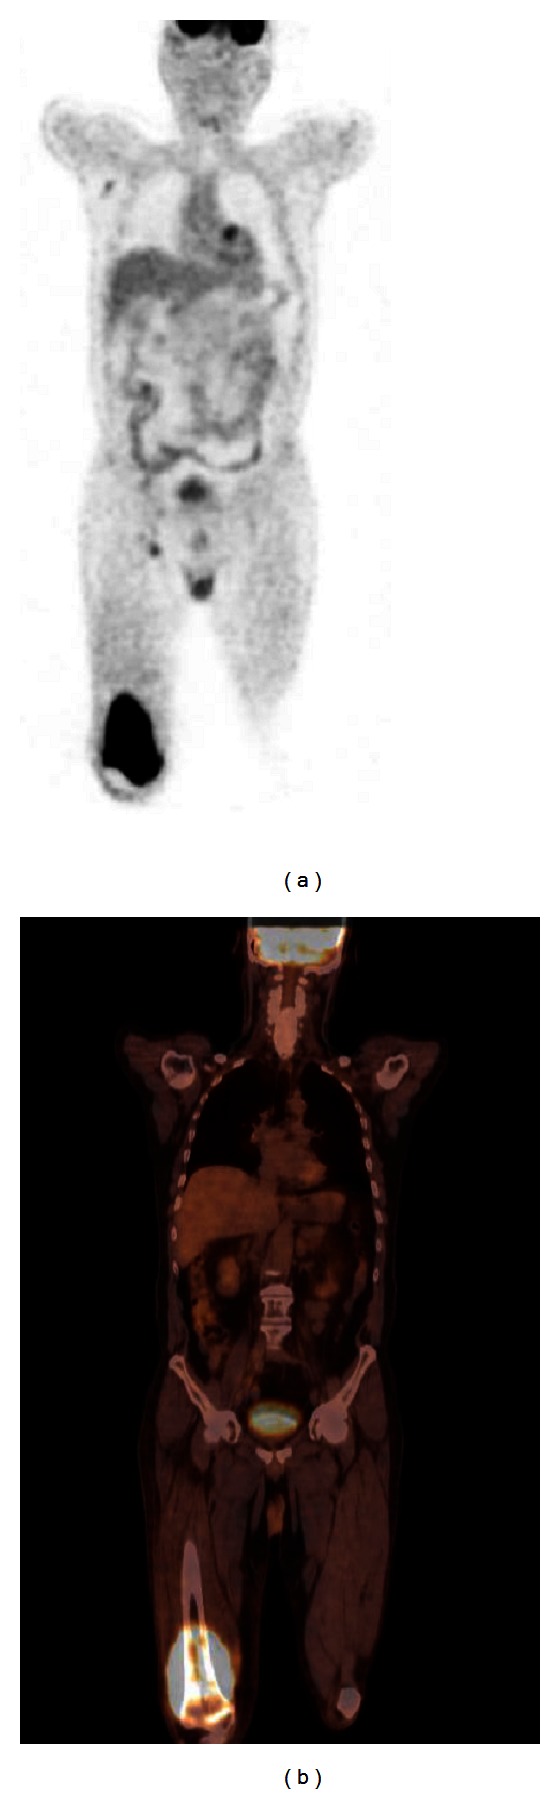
Hypermetabolic lesion, which begins from the middle part of the right femur and invaded the muscular tissue, as well as the bone tissue and bone marrow in the distal diaphysis of the femur, is observed on the PET (a) and fusion (b) images of the patient (SUVmax = 16.1).
